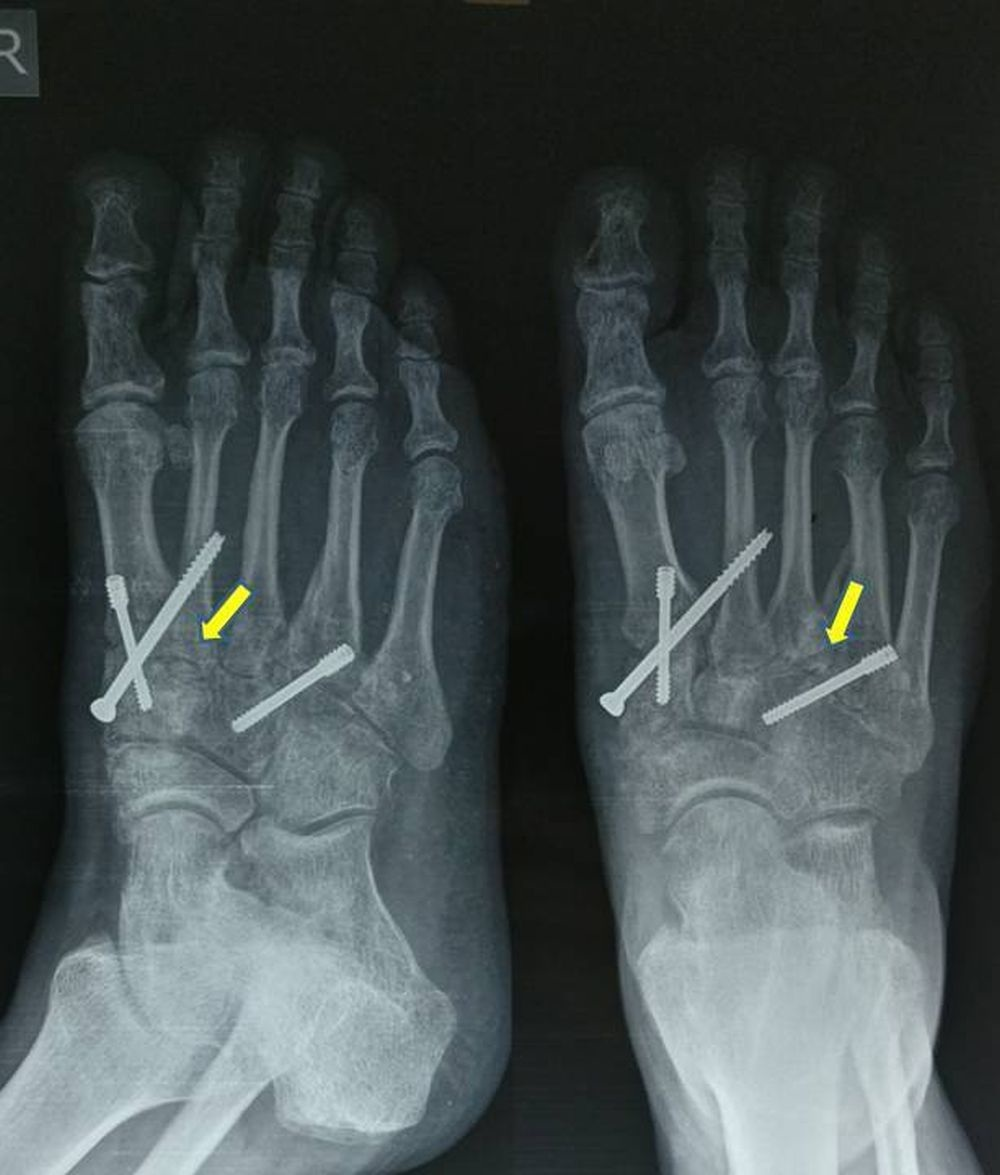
Follow-up radiographs at two months Shows fixation of first, second, and fourth TMT joints is well maintained after removal of the plaster cast.
